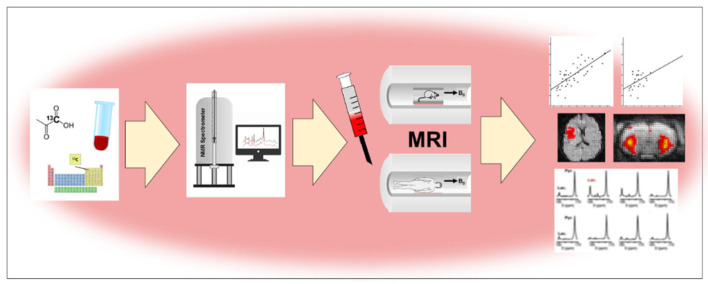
The scope of use of ^13^C MRI in research.
